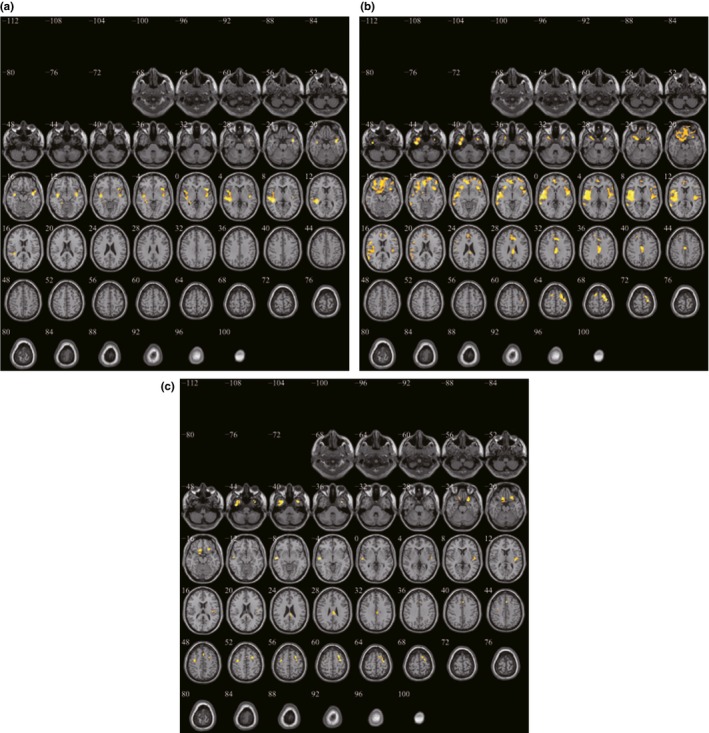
Detected by MRI using SPM8‐VBM8 method, the area of cerebral GM volume reduction in (a) svMCI patients compared with healthy controls, (b) SVaD patients compared with healthy controls, and (c) SVaD patients compared with svMCI patients are shown in yellow color at axial position (FDR‐corrected *p *<* *.05 at cluster level). Age, gender, and educational level were used as covariates. Lighter area represents region with a greater statistical difference
